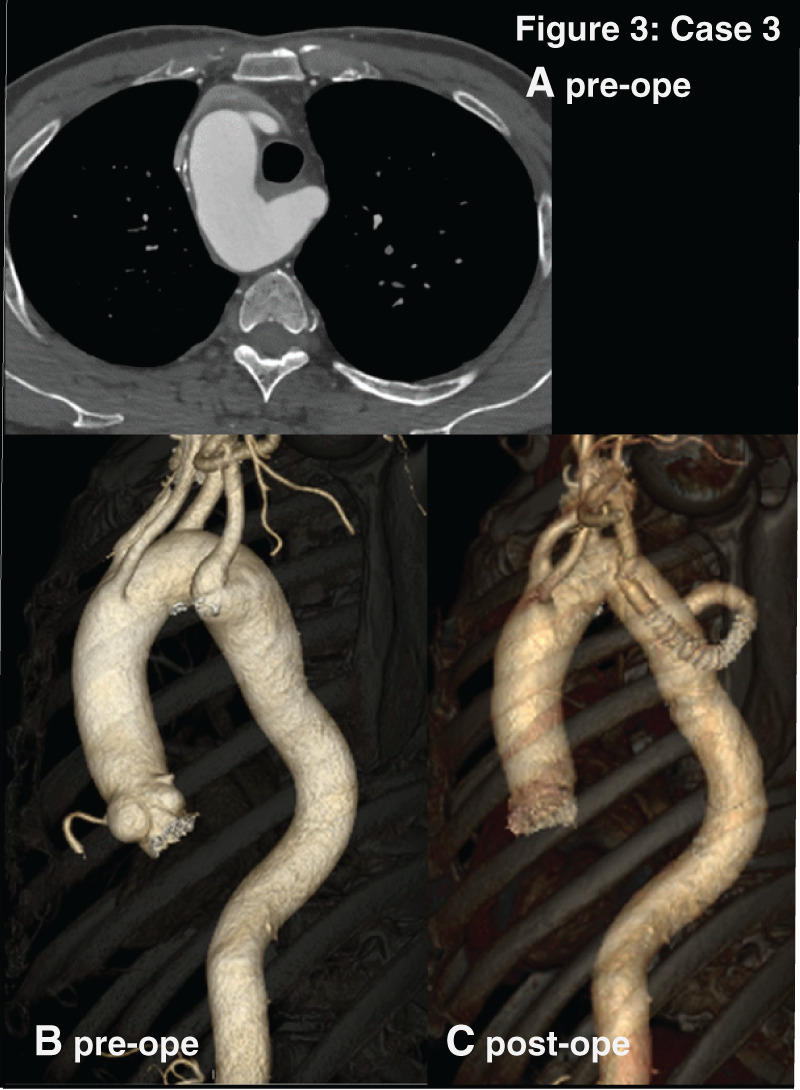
The figure 3 correspond to the images of Case 3 respectively. (A) Preoperative enhanced CT images, axial view. (B) Preoperative 3D-reconstructide CT. (C) Postoperative 3D-reconstructed CT. Case 3 are mirror-image RAAs with ALSCA. ALSCA, aberrant left subclavian artery; RAA, right-sided aortic arch
